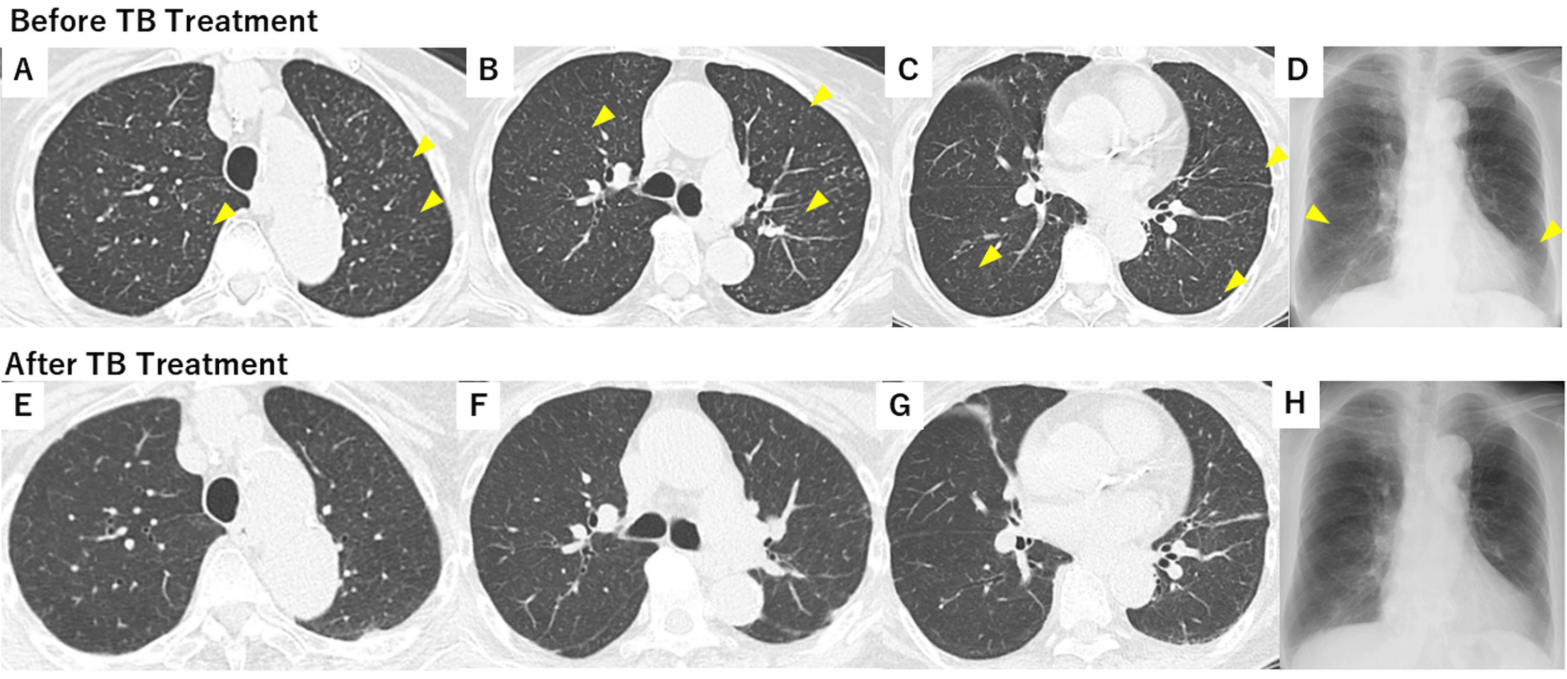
Chest CT and X-ray image before and after anti-TB treatment Chest CT and X-ray images were obtained one and a half months before admission (A-D) and five months after anti-TB treatment (E-H). CT slices were shown at the apex (A, E), pulmonary hilum (B, F), and lower lung field (C, G). Miliary nodules are distributed throughout the lungs (A-D), and only a portion of the numerous nodules are indicated by arrowheads. Those nodules have almost completely disappeared after anti-TB treatment (E-H). CT: computed tomography, TB: tuberculosis
